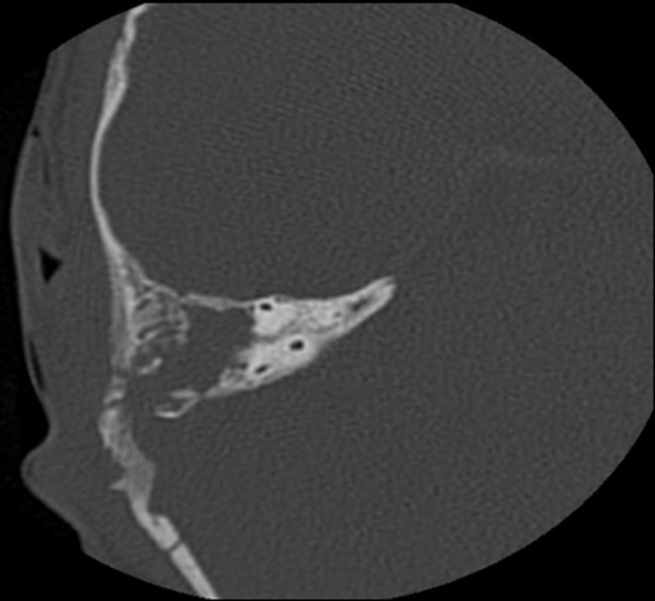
computed tomography scan of the craniofacial region showing bony lysis of the temporal bone and mastoid cells
